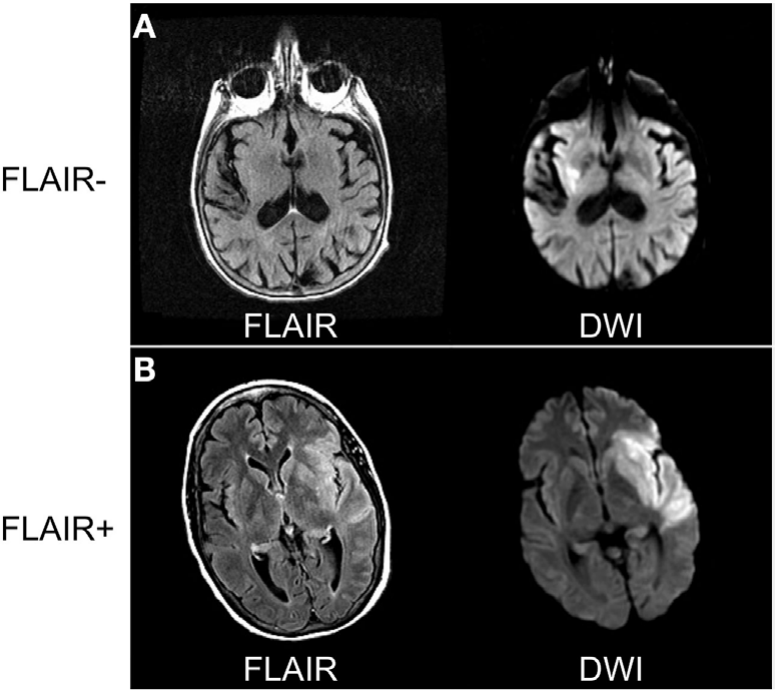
Comparing diffusion-weighted imaging (DWI) and FLAIR sequences to determine radiographic time of stroke onset. (A) Eighty-one-year-old woman awoke with dysarthria and right-sided weakness. Magnetic resonance imaging (MRI) performed 8 h from last-known well (LKW) shows signal abnormality in DWI but not FLAIR sequences. (B) Sixty-three-year-old woman developed sudden onset right-sided weakness with confirmed LKW. MRI performed 5 h from LKW shows signal abnormality on both DWI and FLAIR sequences consistent with stroke onset > 4.5 h. Data analysis for figure was created under approval of local ethics committee.
